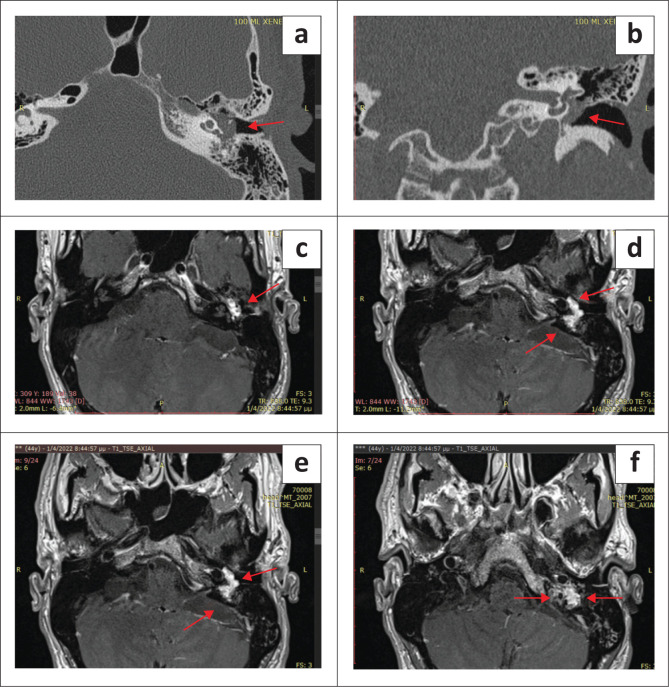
Glomus jugulotympanicum in a 44-year-old female patient. (a,b) Axial and coronal CT images demonstrating the lesion involving the jugular bulb and middle ear, classified as Grade 1 according to Glasscock–Jackson. (c,d,e,f)Contrast-enhanced MRI images of the same patient demonstrating the soft tissue extent of the intense contrast-enhancing tumour, highlighting its vascular nature (red arrows).
